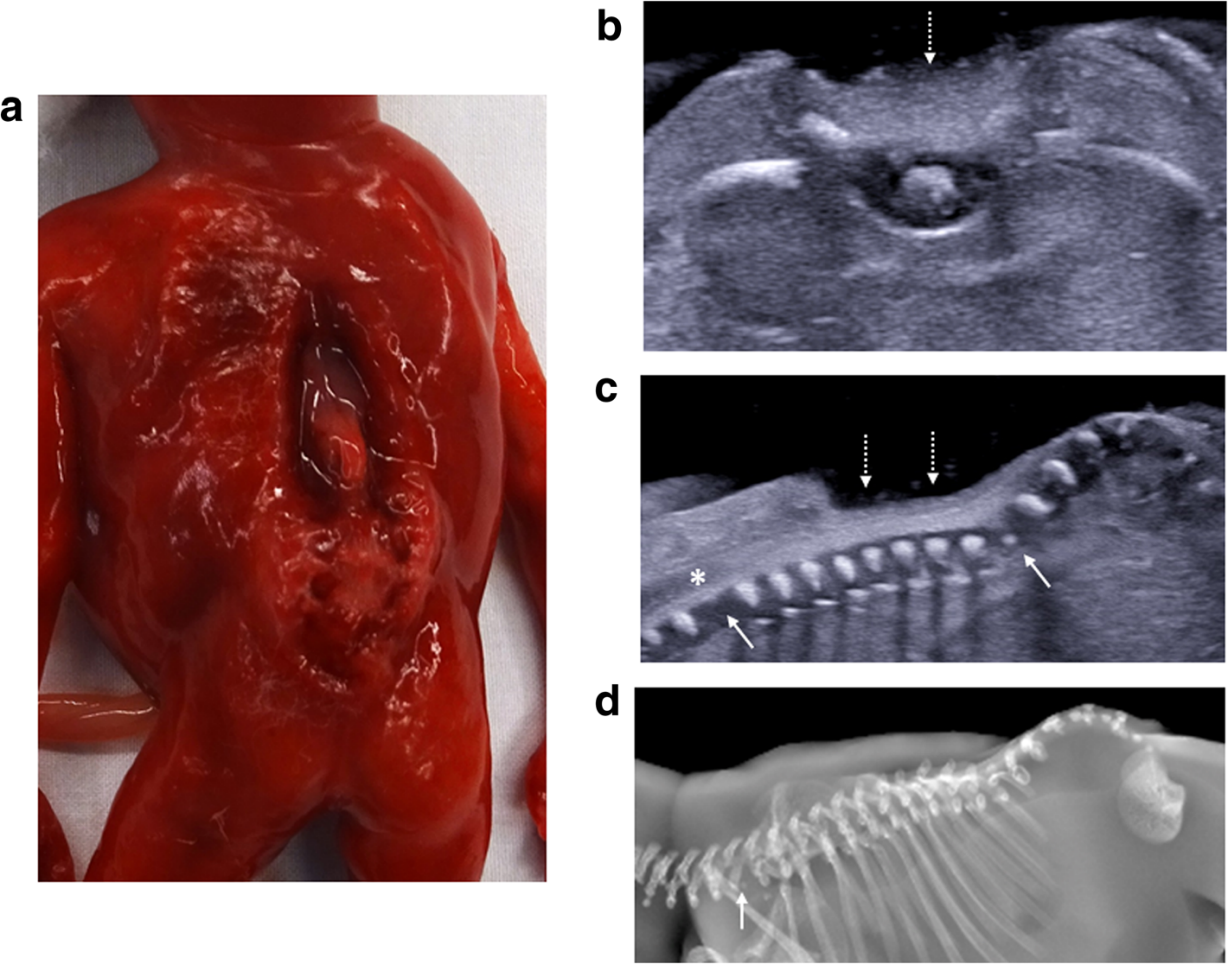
Neural tube defect following termination of pregnancy in a foetus at 16 weeks gestation. On external examination at autopsy (a), the large neural tube defect is readily appreciated. The corresponding post-mortem ultrasound images were obtained 4 days after death in transverse (b) and sagittal (c) planes, demonstrating the absence of posterior vertebral elements (dotted arrows), irregular thoracolumbar vertebral ossification (solid arrows) and high conus medullaris of the spinal cord (asterisk). The lateral post-mortem radiograph of the spine (d) was able to demonstrate sacral spinal dysraphism and irregular thoracolumbar vertebral ossification (solid arrow), although these findings were better depicted on ultrasound
